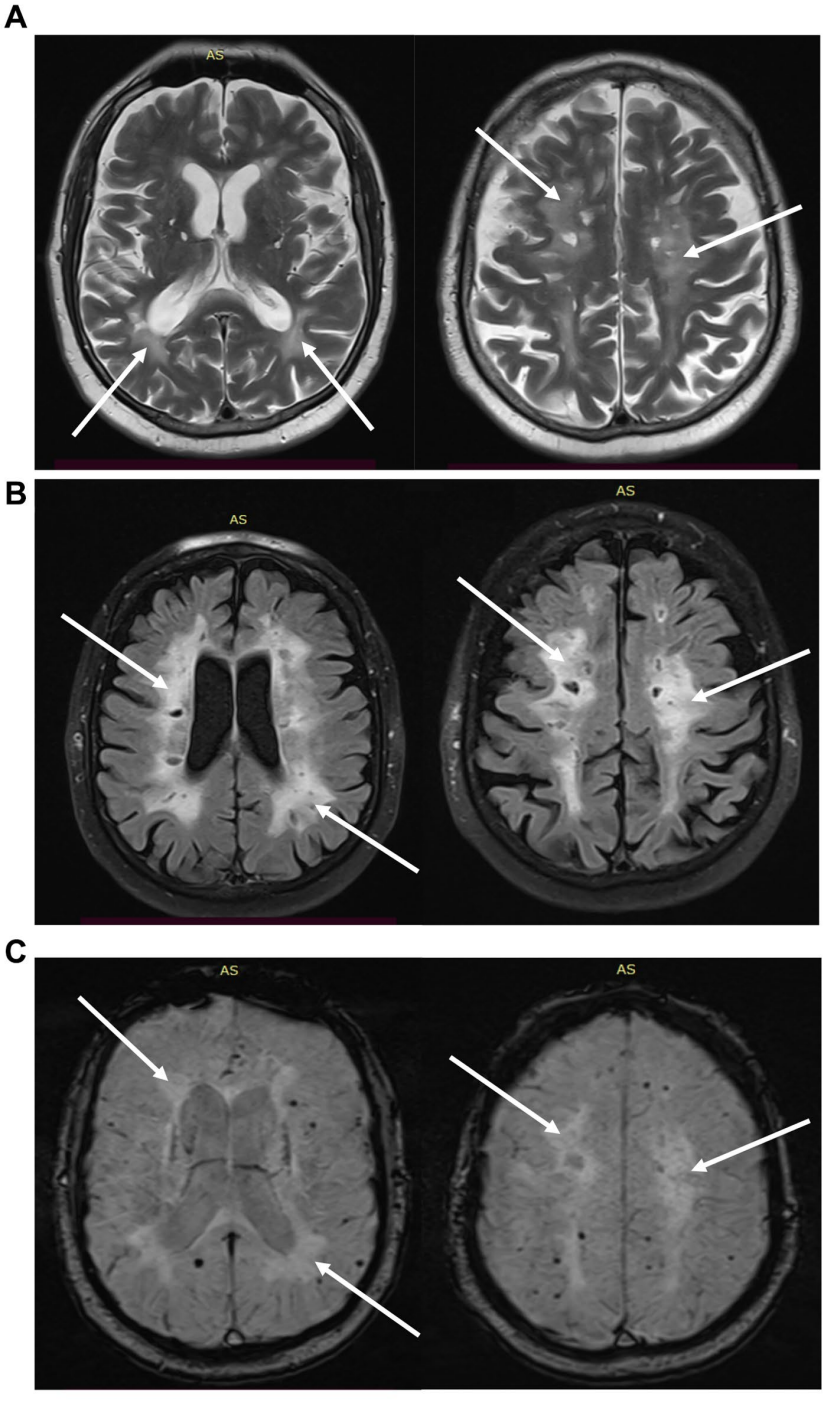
MRI scans of presented MOGAE case showing bilateral T2-hyperintense, poorly demarcated lesions involving supratentorial white matter (solid white arrows). (A) T2-dark fluid MRI, (B) T2-FLAIR MRI, (C) SWI-MRI.
